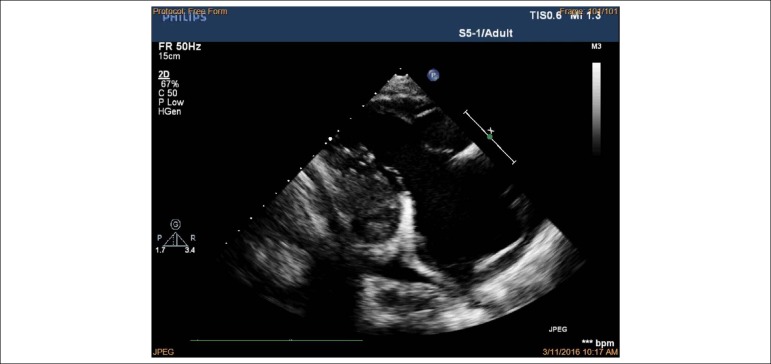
Transthoracic echocardiography from the right ventricular inflow view
